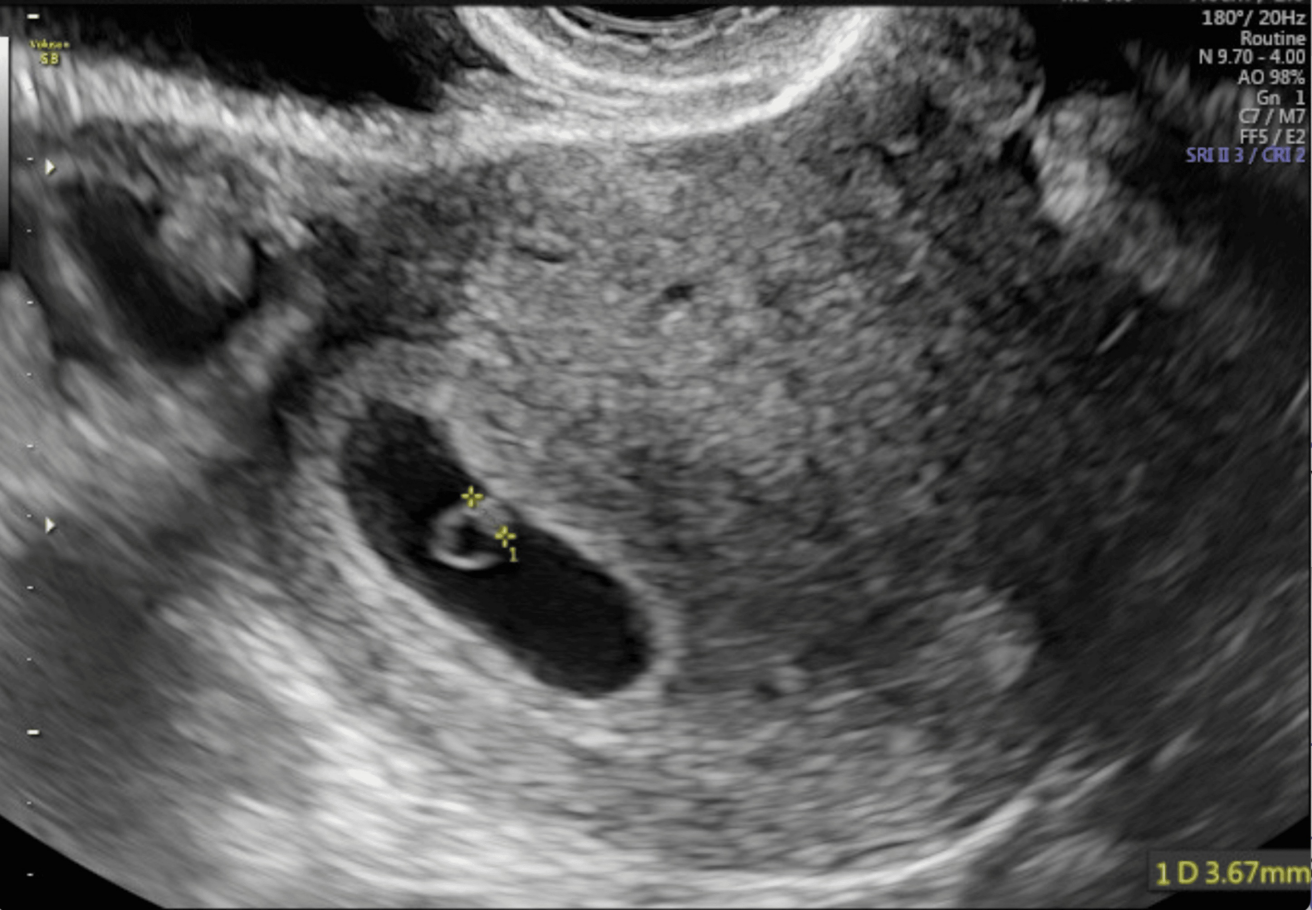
Gestational ultrasound at five weeks + one day.
